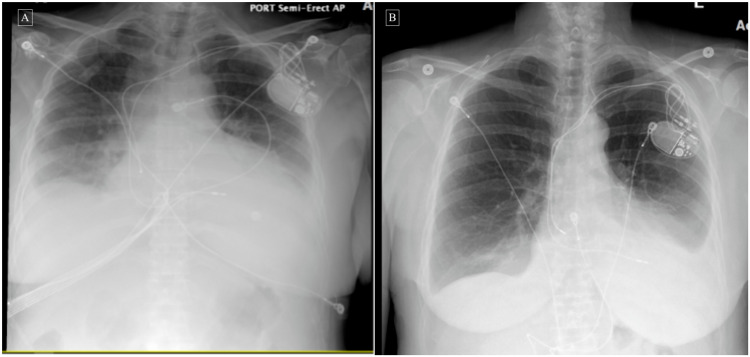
Chest X-rays before and after corticosteroid therapy A: Chest X-ray showing left-sided pleural effusion and/or atelectasis/infiltrate, right-sided pleural effusion, and right basilar atelectasis. B: Chest X-ray after steroids therapy showing decreased left-sided pleural effusion with improved aeration at the left lung base with mild left basal compressive atelectasis and residual effusion, and mild cardiomegaly.
